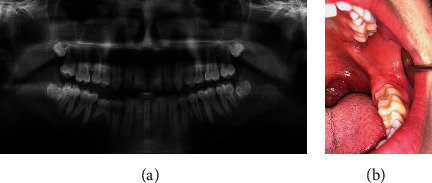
(a) Panoramic radiograph of case #2 prior to third molar extraction showing partially developed and bony impacted #1, 16, 17, and 32. No signs of pathology noted around the impacted #17. (b) Clinical photo post extraction with noted scar tissue at #17 extraction site but otherwise no abnormalities or swelling.
